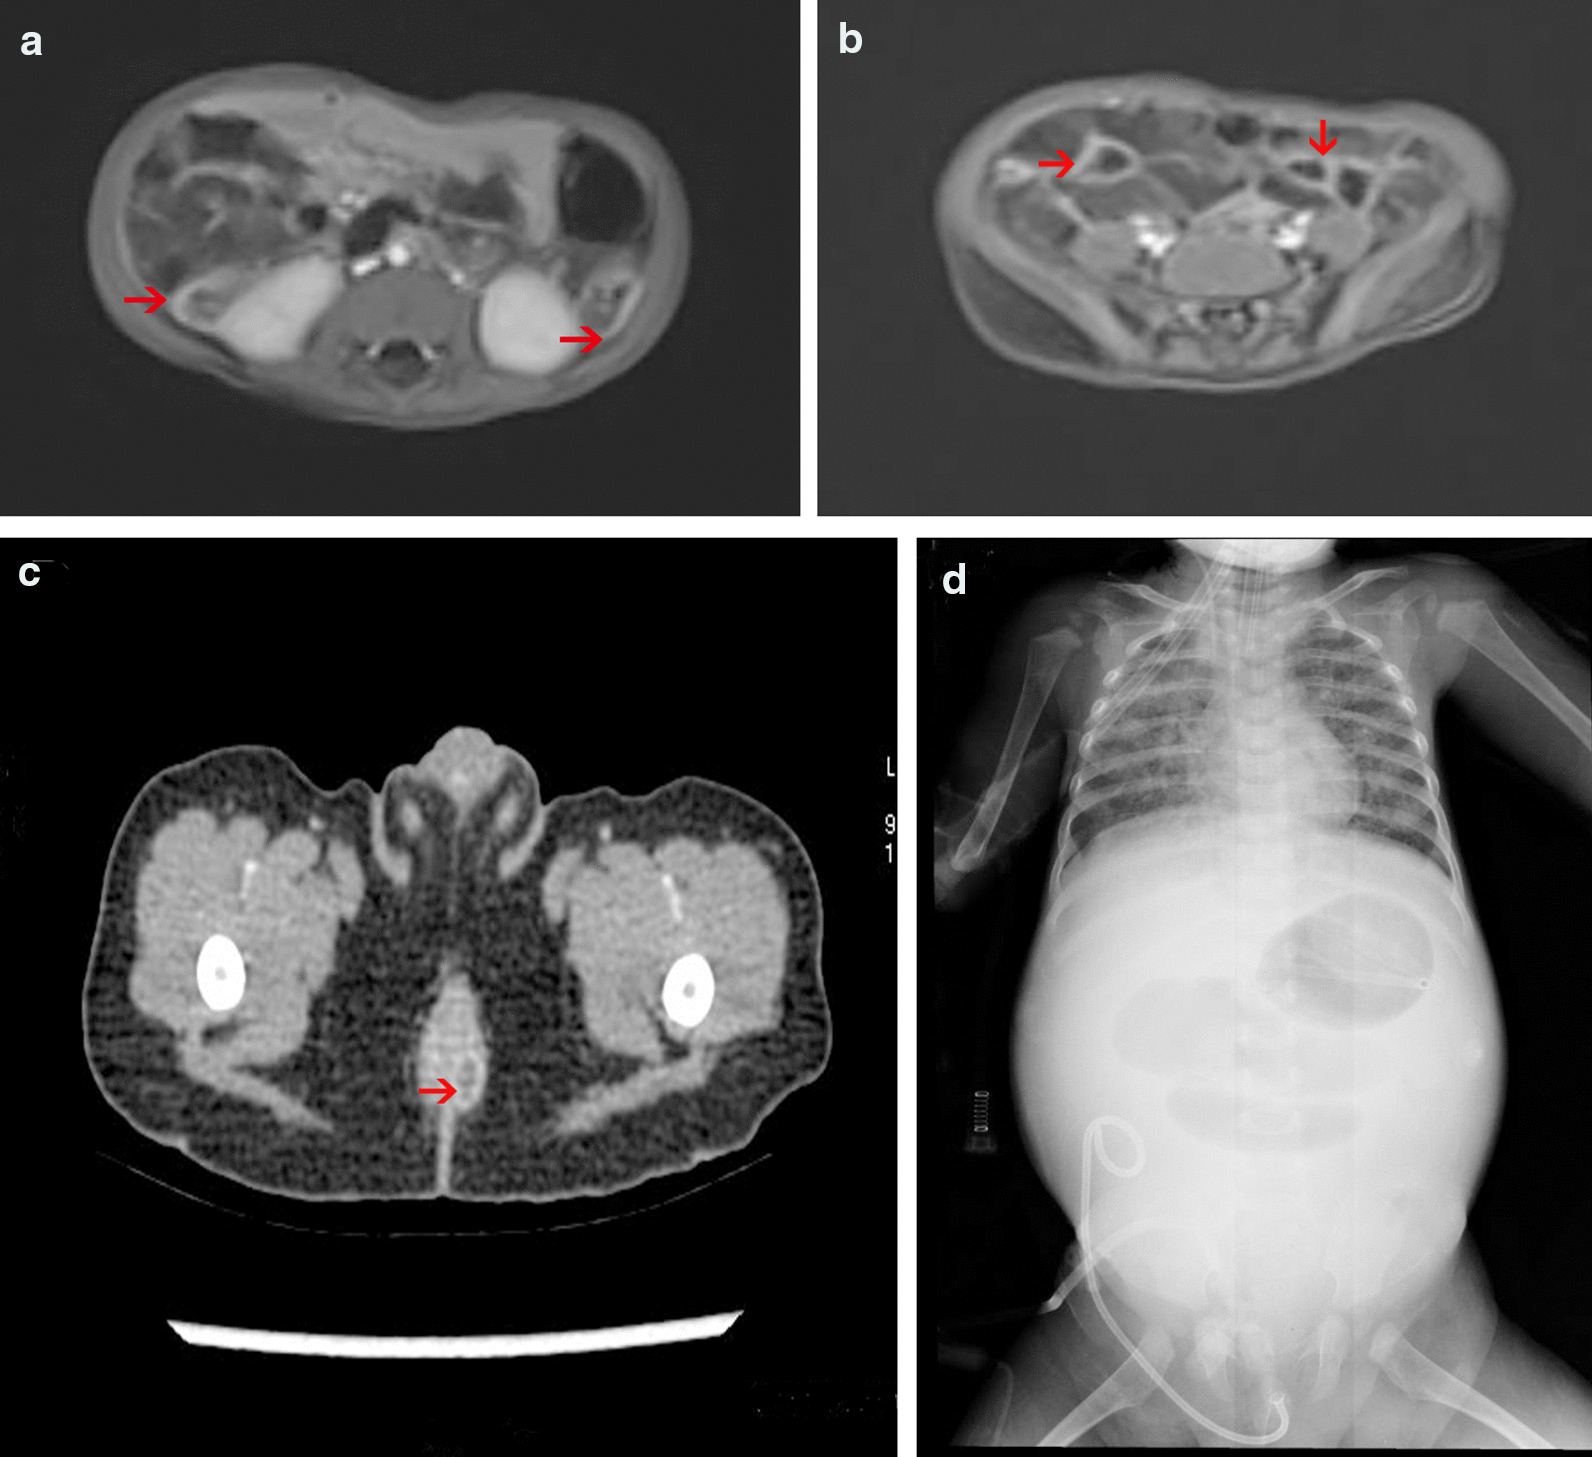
Imaging examination of VEOIBD patients with *IL10RA* gene mutations. a High signal of the colon in the abdominal MRI image of case 1. b High signal of the small bowel in the abdominal MRI image of case 1. c Perianal abscess in the enhanced CT image of case 3. d Intestinal obstruction and necrosis on X-ray examination in case 3
